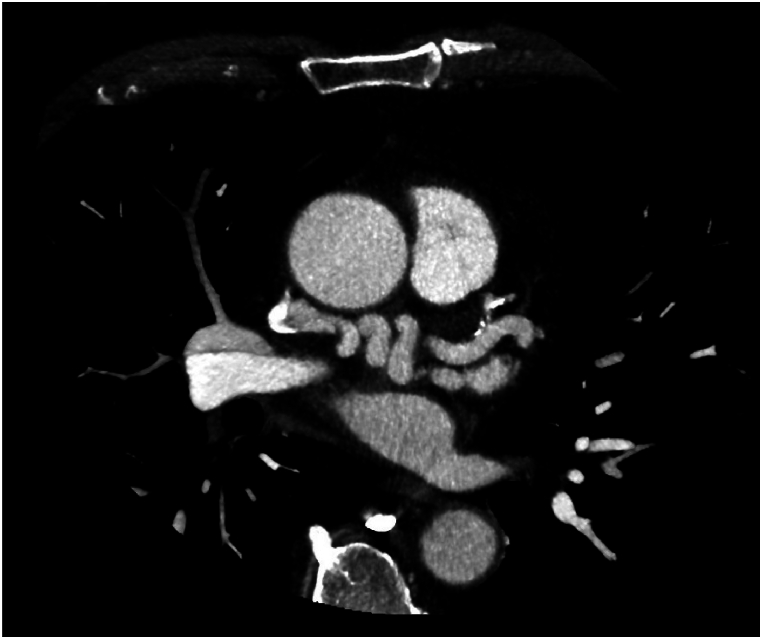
Axial section CT with sinuous fistula circumflex artery and superior vena cava.
